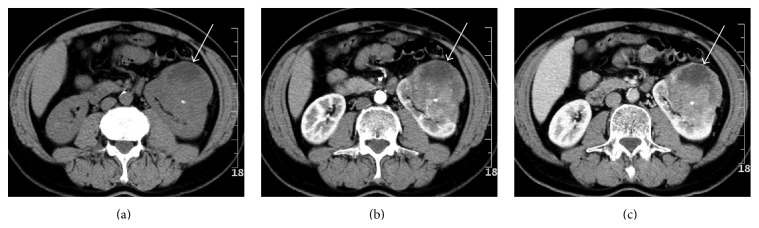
Type 2 papillary renal cell carcinoma in a 58-year-old asymptomatic man. (a) Abdominal plain CT scan shows an irregular ill-defined heterogeneous (around 44 HU) mass of 8.3 cm in size in the left kidney. Note a punctate calcification within the lesion. (b) The tumor, which involves both the cortex and medulla, shows heterogeneously moderate enhancement (to around 80 HU) during corticomedullary phase. (c) The tumor's attenuation remains around 80 HU in the nephrographic phase. The unenhanced areas within the tumor indicate necrosis.
